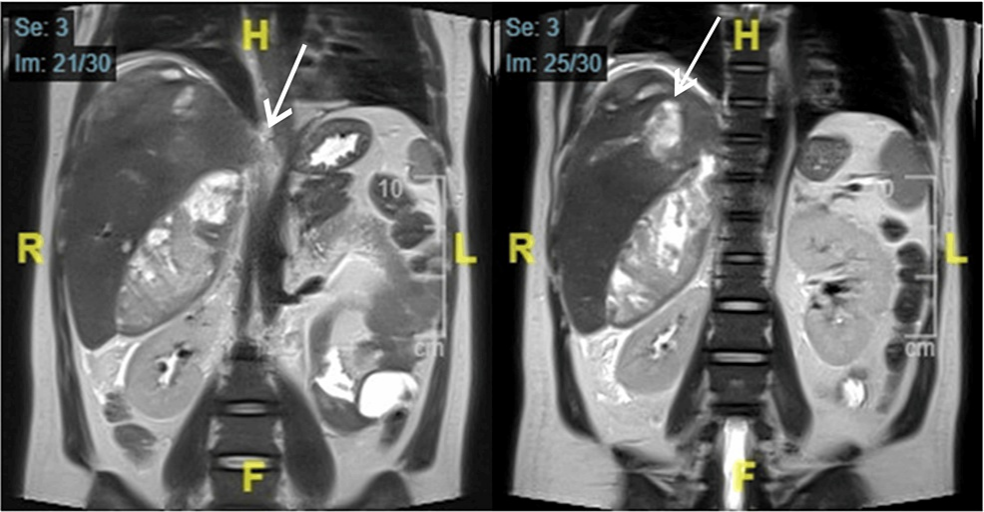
Coronal magnetic resonance imaging of the liver/magnetic resonance cholangiopancreatography showing a newly developed subcapsular collection. The left hepatic lobe was atrophied, with dilated bile ducts and multiple intraductal stones.
